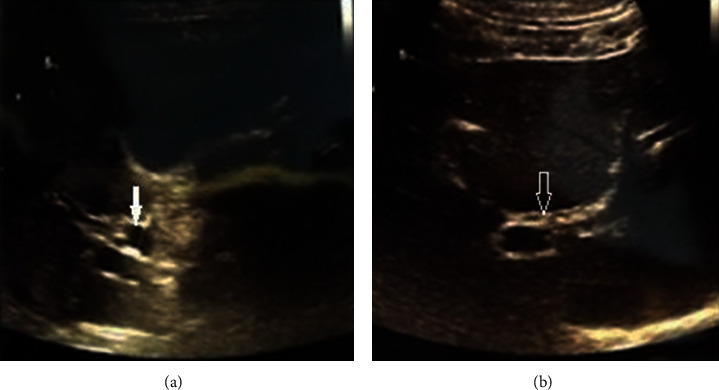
Abdominal ultrasound showing (a) dilated common bile duct (white arrow) (b) on the 12th hospital day normal appearance of common bile duct (arrow).
